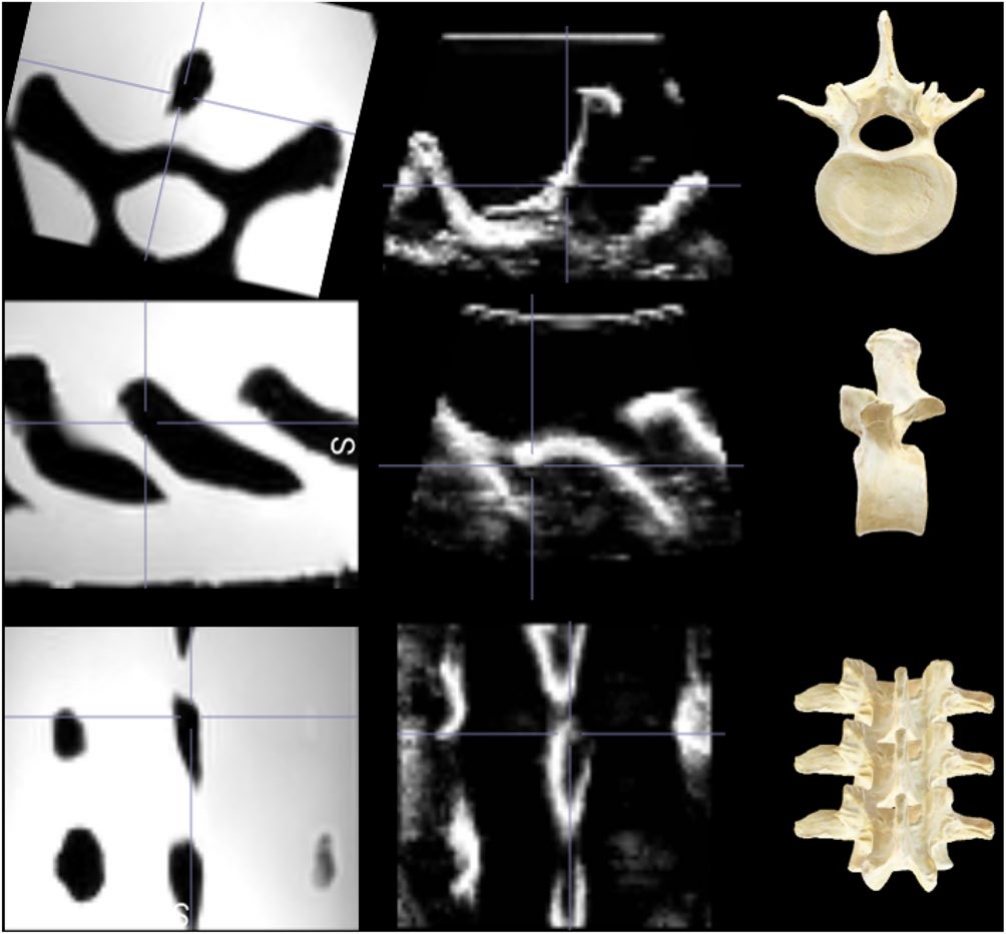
The transverse (top row), sagittal (middle row), and coronal (bottom row) views are shown of the processed US and MRI volumes used for training the algorithm. Each column from left to right shows the MRI, US, and respective anatomical model views. The US and MRI volumes were used to train the GAN algorithm. The anatomical models shown are not representative of the T9 vertebra, instead they are used as visual indicators of the transverse, sagittal, and posterior coronal views.
